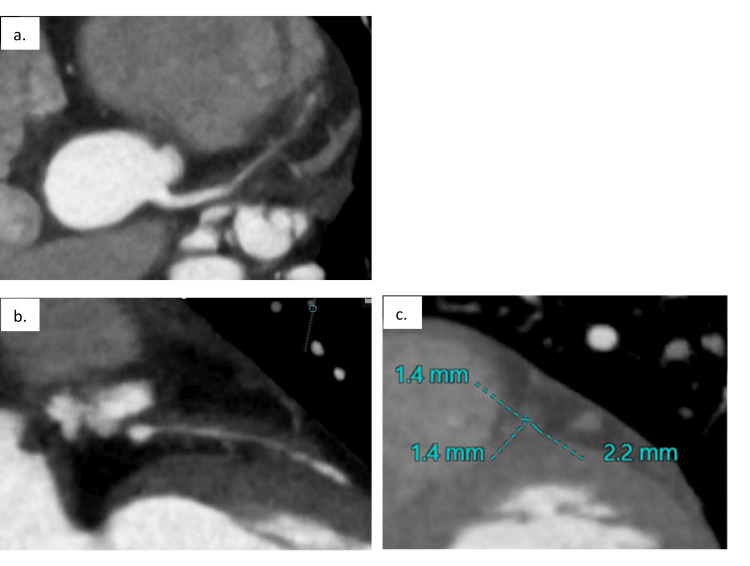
Cardiac CT completed after receiving a cumulative dose of 6,400 mg cyclophosphamide. Compared to the prior CT, the left anterior descending (LAD) artery wall thickness was significantly improved, but the intraluminal diameter was unchanged (a, b). The LAD diameter remained <2 mm, and the surrounding wall thickness measured 2.2 mm (c).
